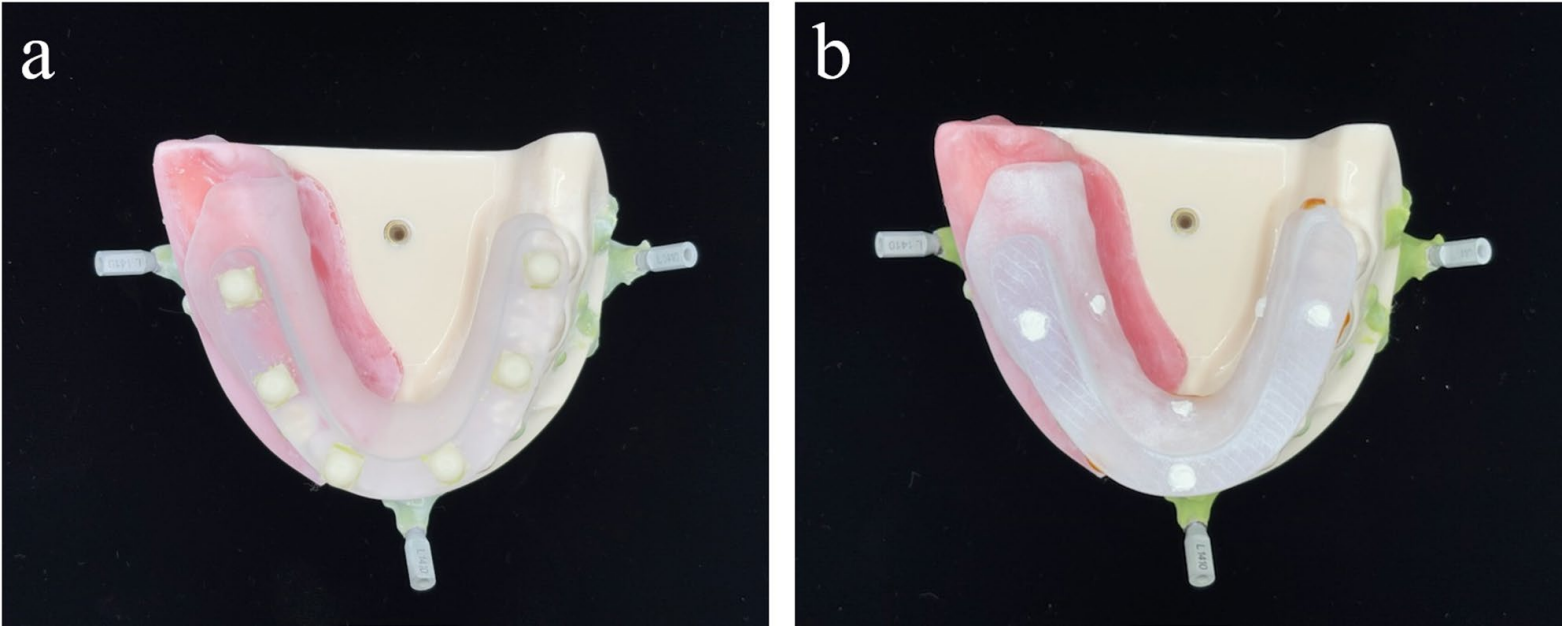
Fabricated CT matching templates (CTMTs). a GCCTMT mounted on the master model. b GPCTMT mounted on the master model
